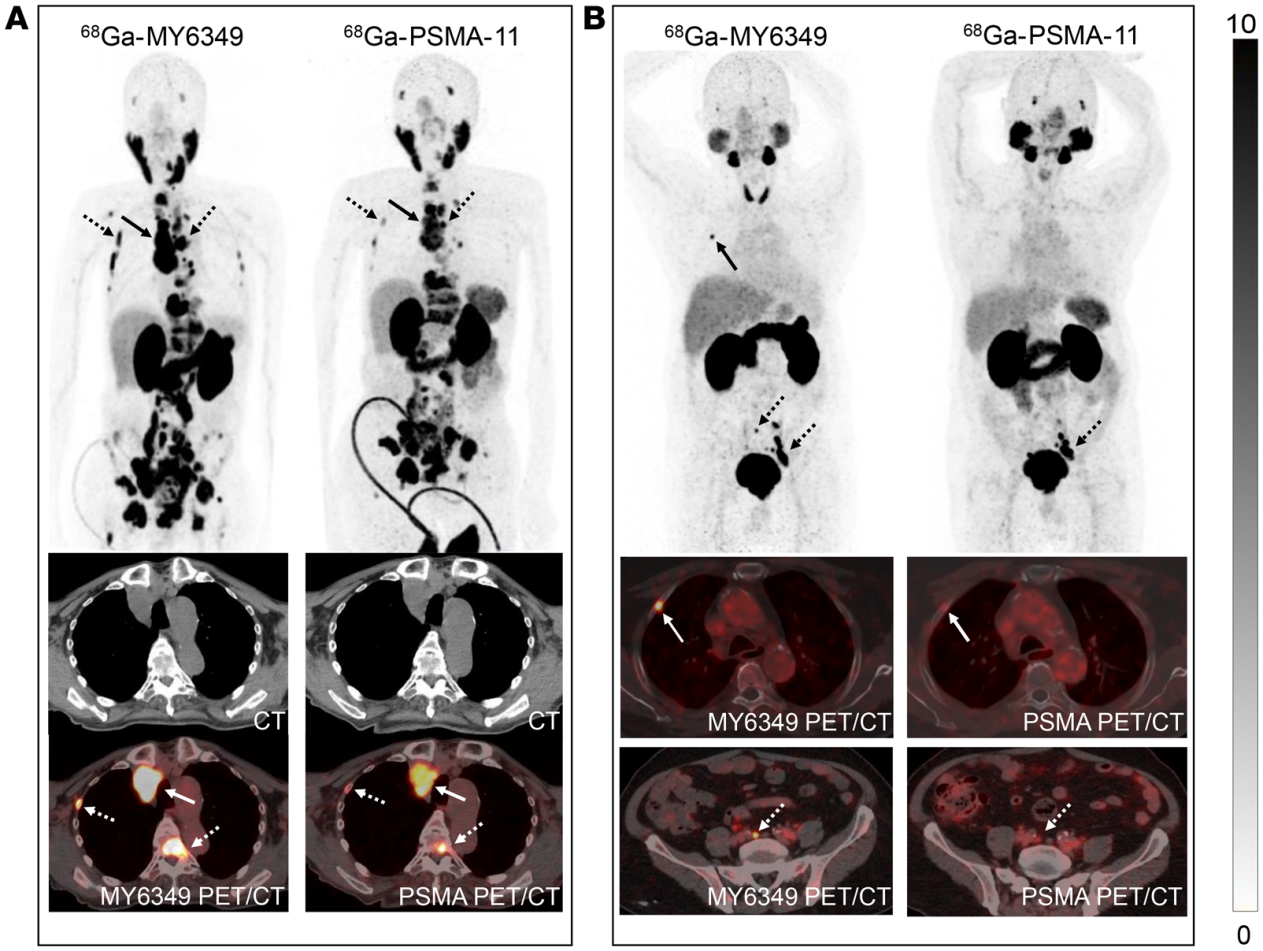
Representative ^68^Ga-MY6349 PET/CT and ^68^Ga-PSMA-11 PET/CT images in patients with metastatic prostate cancer. (A) A 77-year-old man with prostate cancer who had received endocrine therapy for 3 years underwent PET/CT imaging for restaging. Compared with ^68^Ga-PSMA-11 PET/CT, ^68^Ga-MY6349 PET/CT revealed more metastatic lesions and exhibited higher tracer uptake in the lymph node metastasis (solid arrows) and bone metastases (dotted arrows). (B) A 70-year-old man with pathologically confirmed prostate cancer underwent PET/CT for initial staging. ^68^Ga-MY6349 PET/CT demonstrated higher tracer uptake in the bone metastasis (solid arrows) and the involved lymph node (dotted arrows) than that of 68Ga-PSMA-11 PET/CT.
